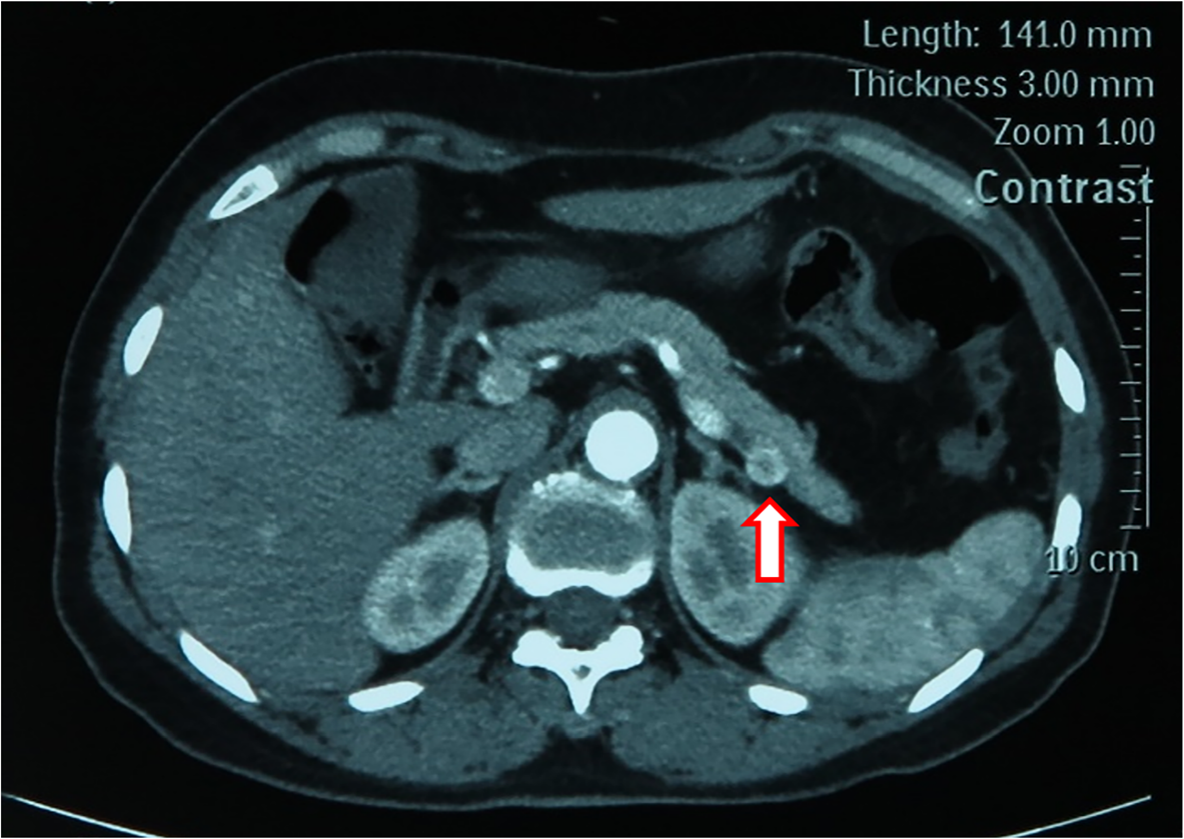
Contrast-enhanced CT showing a tumor located in the dorsal portion of pancreatic tail, close to splenic vessels. The arrow indicates the tumor
